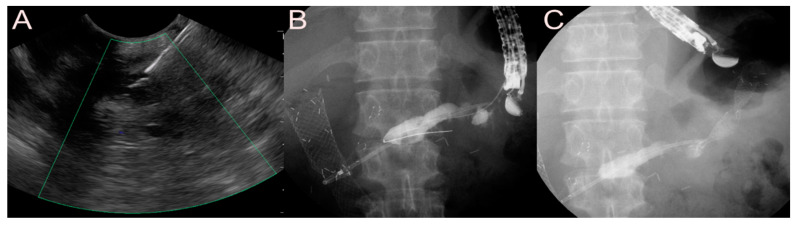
Endoscopic ultrasound-guided pancreatic duct (PD) drainage using the bi-flanged fully covered self-expandable metal stent (FCSEMS) (BONA-AL Stent; Standard Sci Tech Inc., Seoul, Korea) for obstructive pancreatitis. (A) Endosonographic image showing a 19-gauge needle puncture into the dilated main PD. (B) Fluoroscopic image showing a guidewire delivered to the head portion of the main PD through a 19-gauge needle punctured into the upper site of the stomach. (C) Fluoroscopic image showing the bi-flanged FCSEMS placed between the main PD and the stomach.
